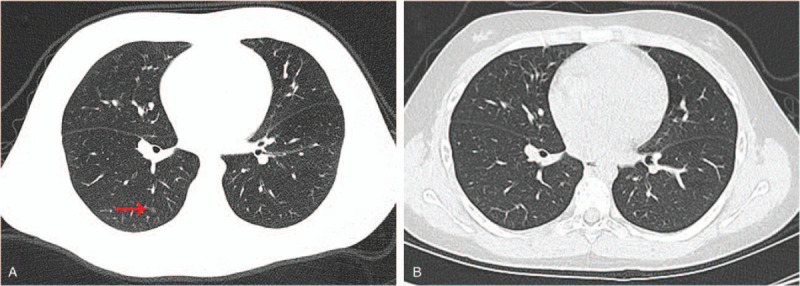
Chest CT results of the moderate case. The 2 results were separated by 7 days. The lesion in the lower lobe of the right lung was indicated by the red arrow.
